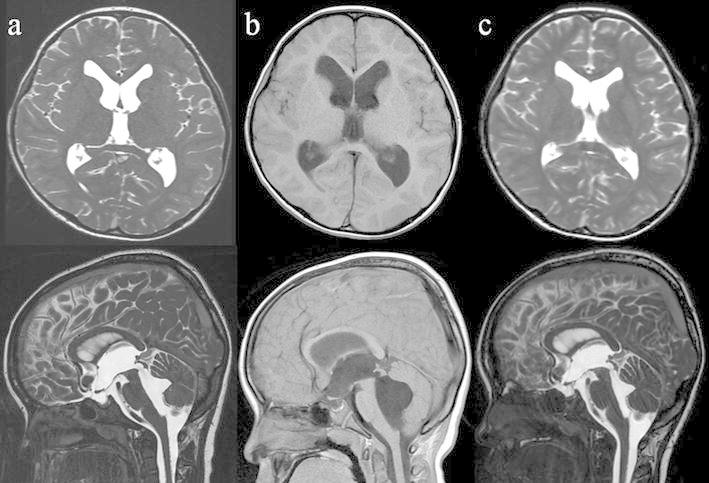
a Magnetic resonance imaging (MRI; fast imaging employing steady state acquisition, FIESTA), performed 1 month after the surgery, shows significant shrinkage of each ventricle. b MRI (T1-weighted image), performed 1 year after the initial endoscopic third ventriculostomy (ETV), indicating recurrent hydrocephalus. c MRI (FIESTA), performed 6 months after the second ETV, indicating improvement of the hydrocephalus
